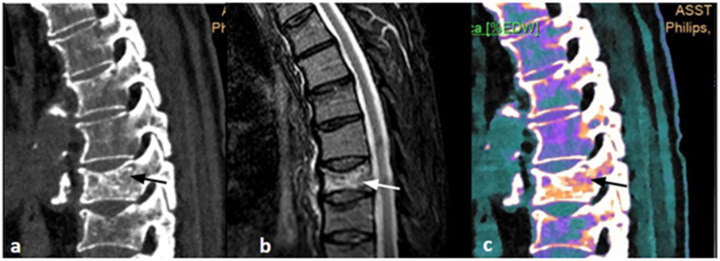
(a) Sagittal CT image, baseline acquisition of the thoracic spine, showing only the osteodense aspect of the vertebral body of D8 (black arrow). (b) Sagittal MRI acquisition with STIR sequence, clearly showing the hyperelectronicity of the D8 vertebral body, indicative of interstitial edema (white arrow) and an acute process. (c) Sagittal DL-SCT image, post-processed with IntelliSpace software, showing an increase in ED at the level of D8 vertebral body (orange color, black arrow), indicative of spongy edema. Color bar legend. White: normal cortical bone; orange: intermediate to high ED; purple: low ED; blue: very low ED, consistent with normal bone marrow composition rich in fat.
